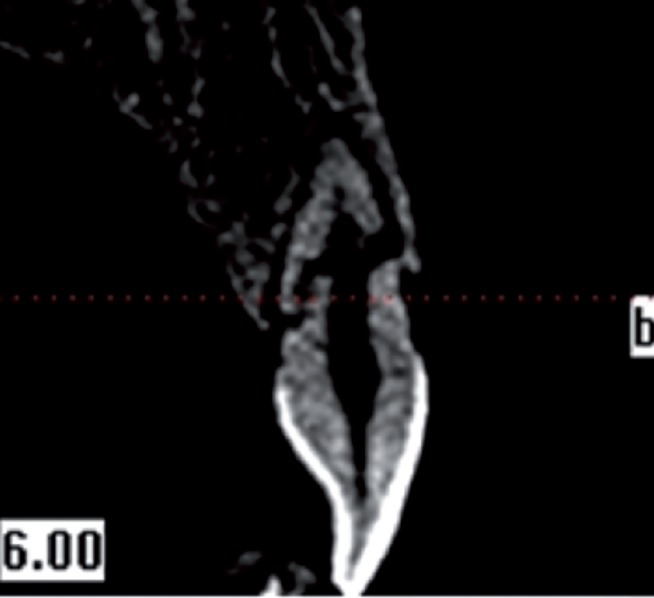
Fragmentary root fracture CBCT view.
